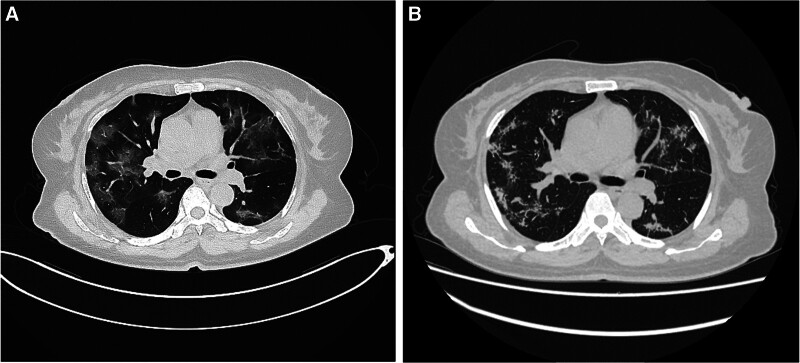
(A) The initial chest CT scan displays patchy infiltrates with extensive patchy areas of ground glass opacification, typical of COVID-19 pneumonia. (B) After 6 weeks from the diagnosis, the Chest CT shows extensive resolution of lung infiltrates, as well as areas of consolidation.
